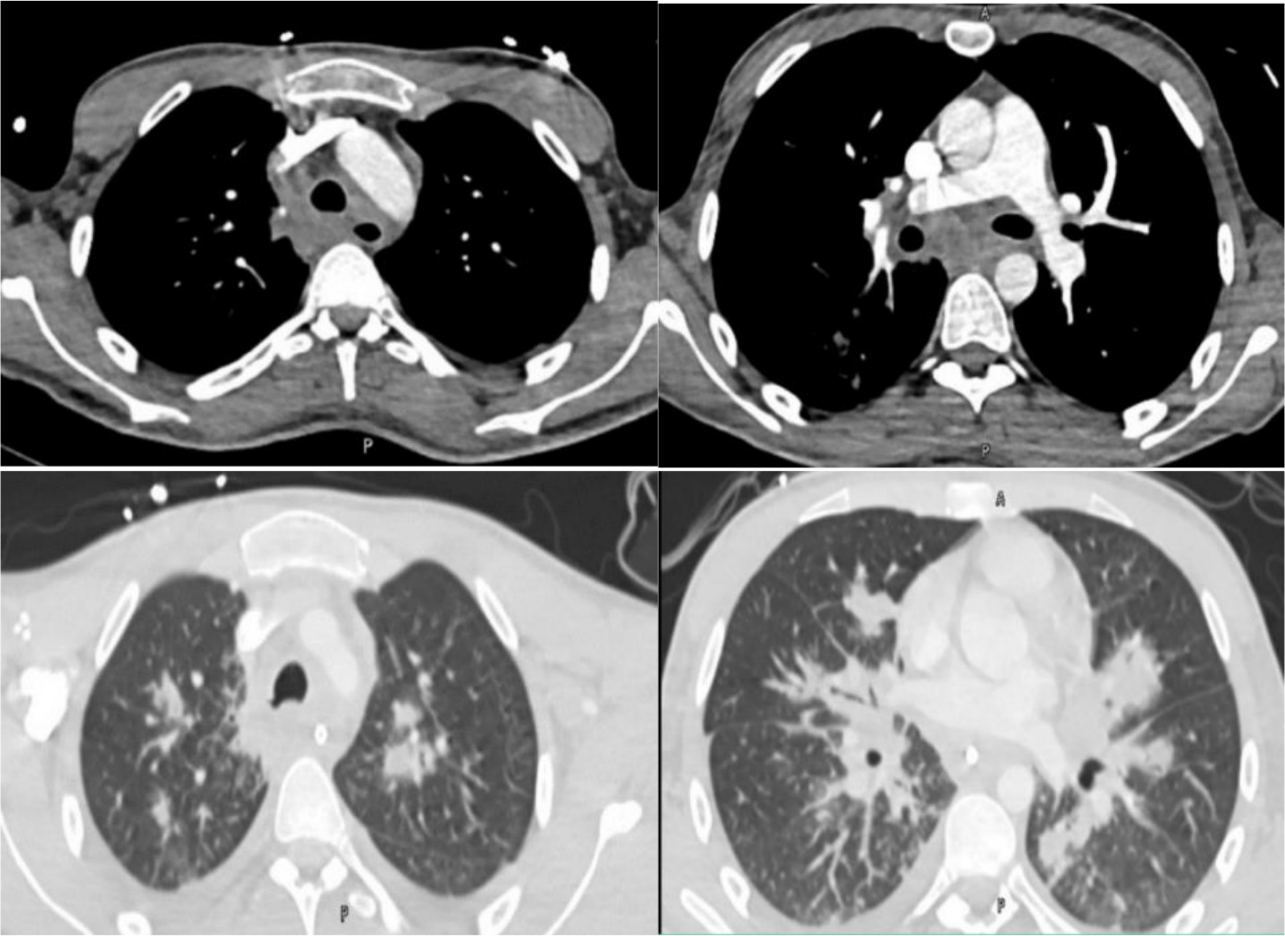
CT pulmonary angiogram shows extensive infiltrative changes in the mediastinum, and lung consolidations suggestive of acute mediastinitis and lung involvement.
